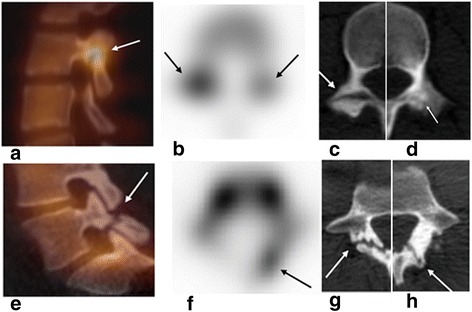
Isthmic spondylolysis: progressive lesion versus terminal stage of chronic nonunited lesion. a Sagittal SPECT/CT image in a 16-year-old female gymnast complaining of lumbar back pain showed intense increased uptake at the right L3 pars defect. Uptake at bilateral L3 pars fractures more prominent on the right is seen in axial SPECT image (b) suggesting progressive lesions more acute on the right; axial CT images at two slightly different levels of L3 vertebra (c, d) show bilateral fracture line defects. e Sagittal SPECT/CT image and axial SPECT image (f) in a 19-year-old female with remote history of cheerleading, presenting with low back pain showed no uptake on the chronic nonunited right L5 pars defect; axial CT images at two slightly different levels of L5 vertebra (g, h) showed fracture line and sclerosis on the right and sclerosis, overgrowth of the contralateral (*left*) posterior elements and lamina fracture due to stress and bony remodeling in an unstable posterior neural arch
